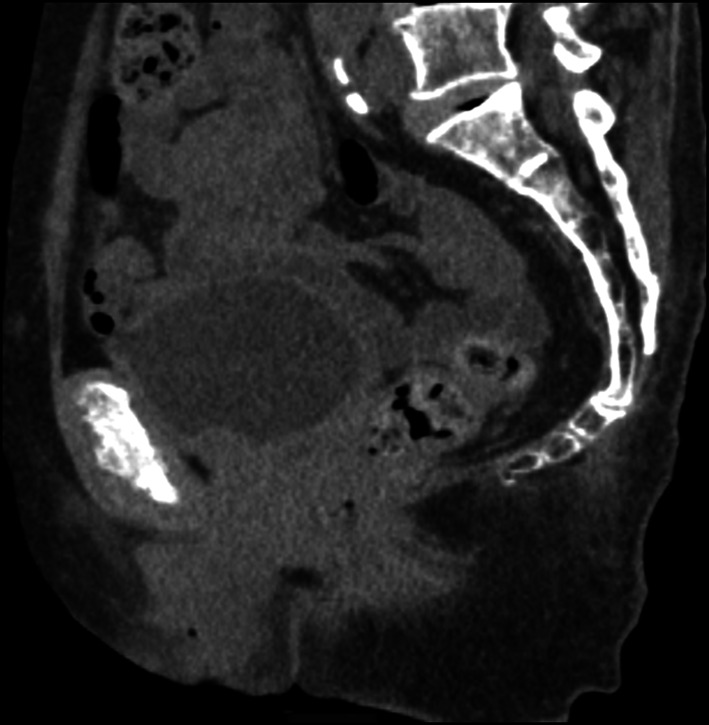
Computed tomography (CT) scan shows no evidence of recurrent rectal prolapse one year later after surgery
